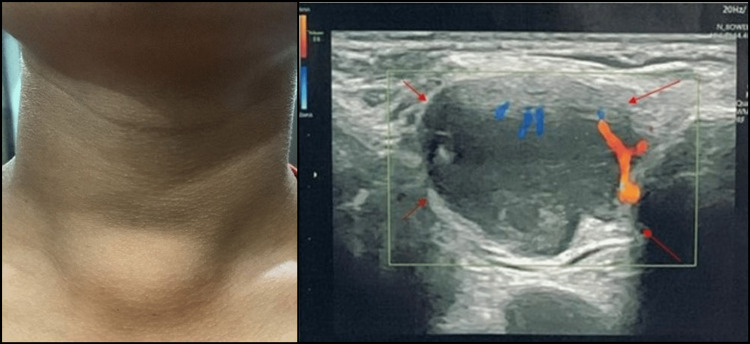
Midline neck swelling and ultrasonography neck: red arrows suggested a hypoechoic lesion of approximately 18 x 33 x 24 mm in the suprasternal region
